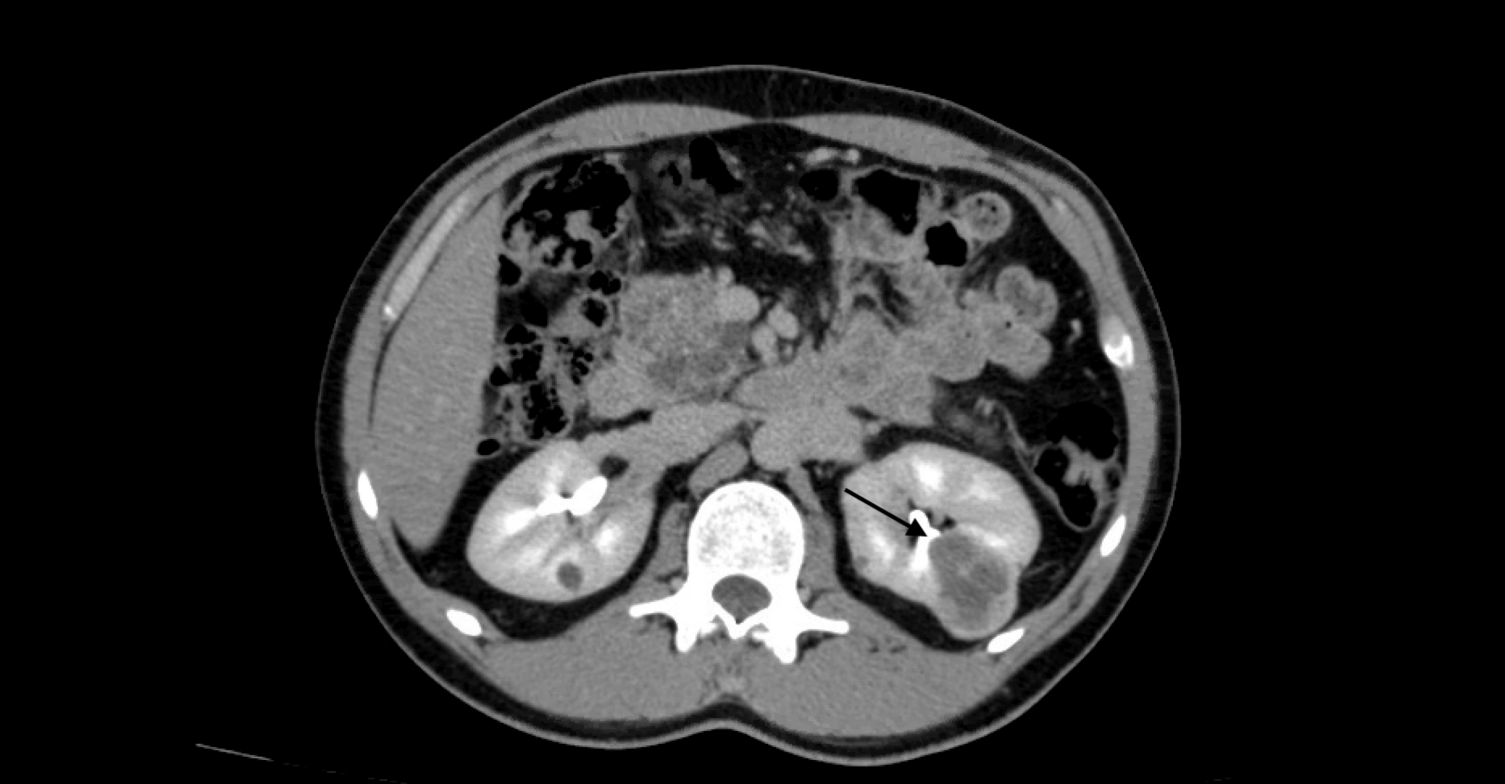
CT urography showing three complexes of multifocal RCC masses (axial view) CT: computed tomography, RCC: renal cell carcinoma
